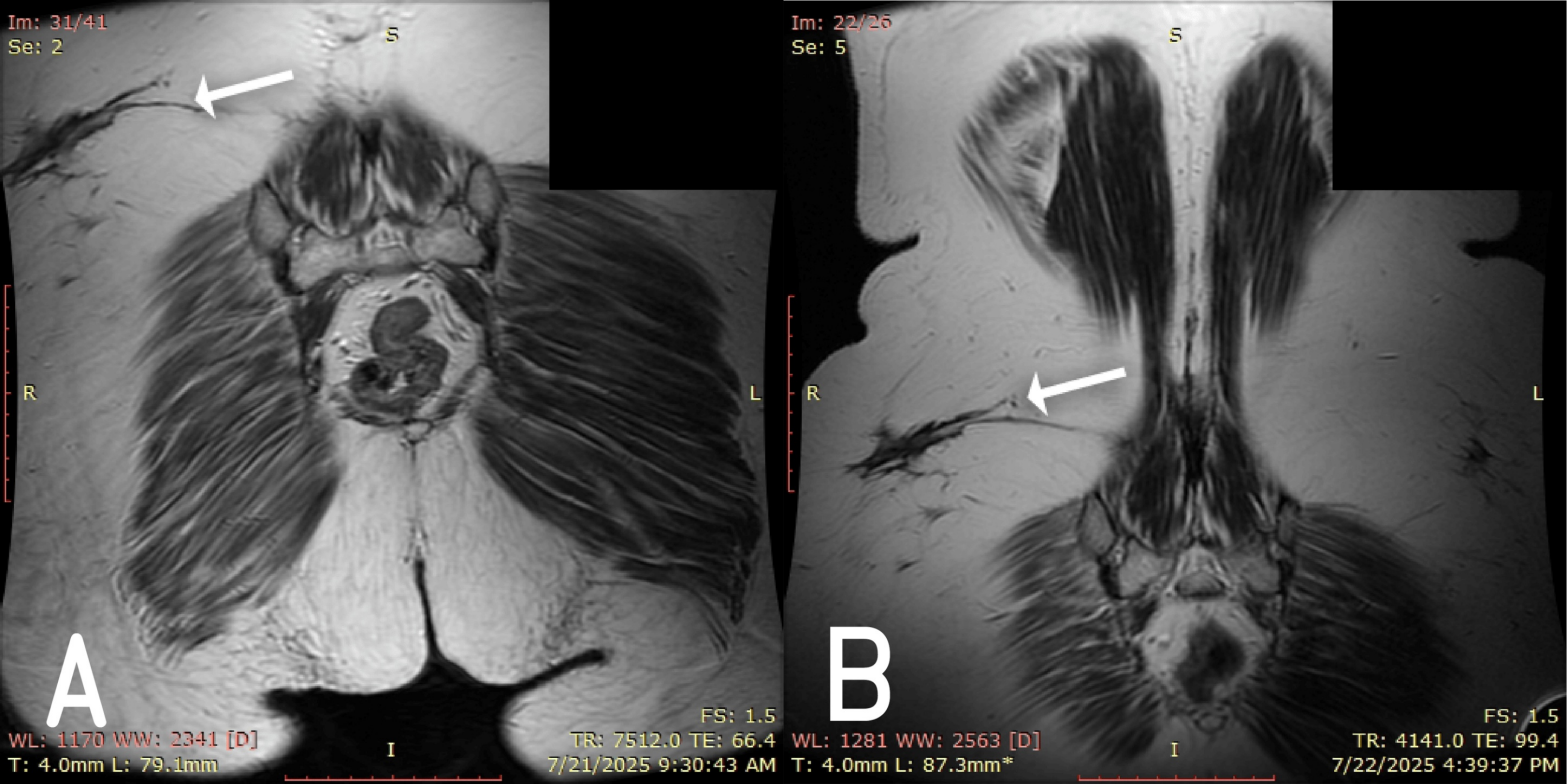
Preoperative pelvic MRI showing fluid accumulation with fistulization to the retroperitoneum (white arrows).
